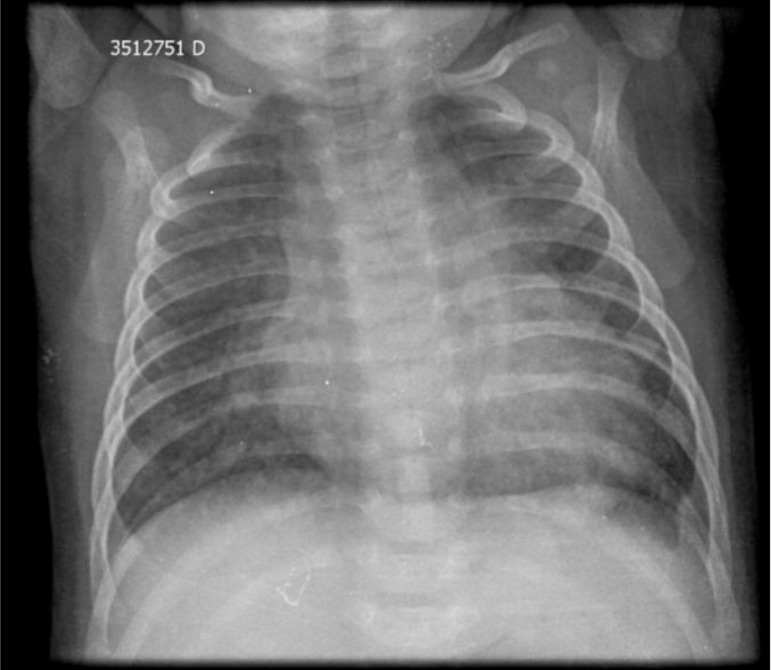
Chest radiography demonstrates cardiomegaly and prominent pulmonary vascular markings.
